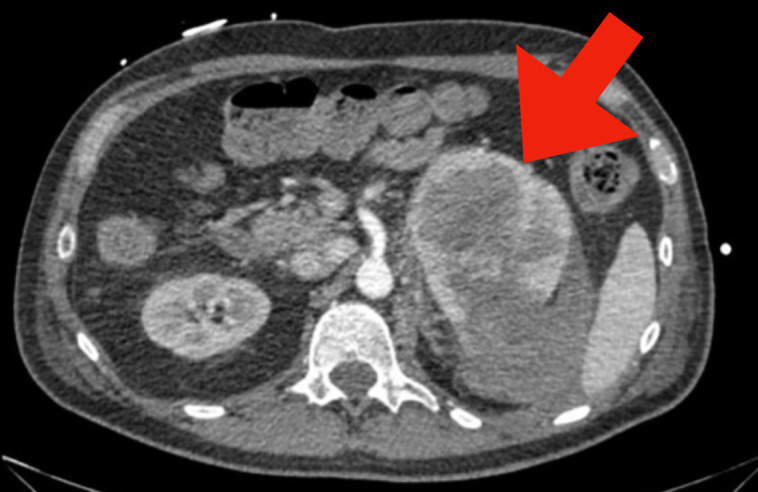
Axial CT angiogram demonstrating ruptured left adrenal mass hematoma with hyper dense blood products extending into the left retroperitoneum and pelvis but no active extravasation. The arrow identifies the lesion of interest. It is important to note the difference in density between the blood product and adrenal gland.
